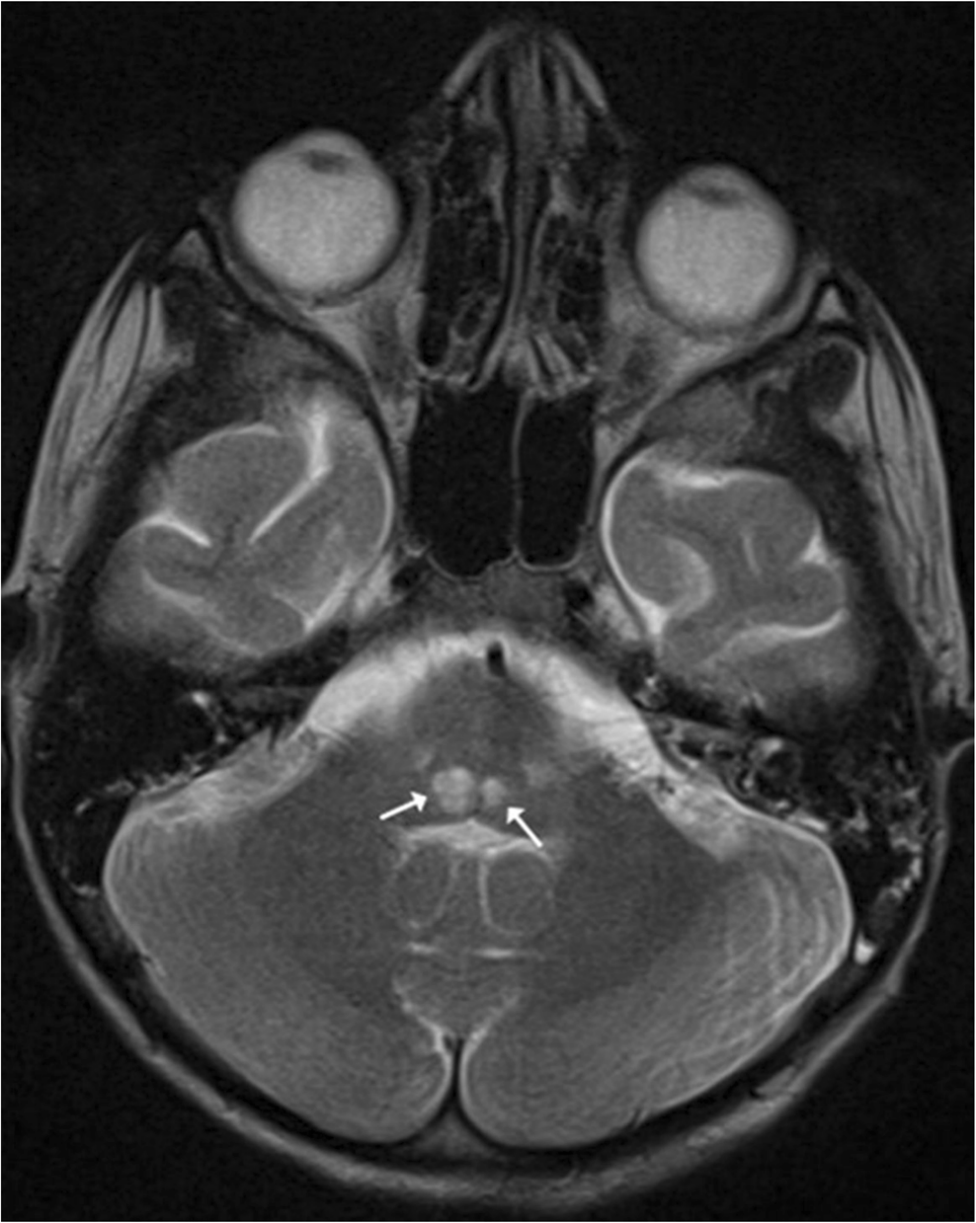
A 3-year-old patient with cerebral palsy and delayed milestones: Axial T2-weighted image reveals focal tegmental hyperintensities at the level of medulla (*arrows*)
